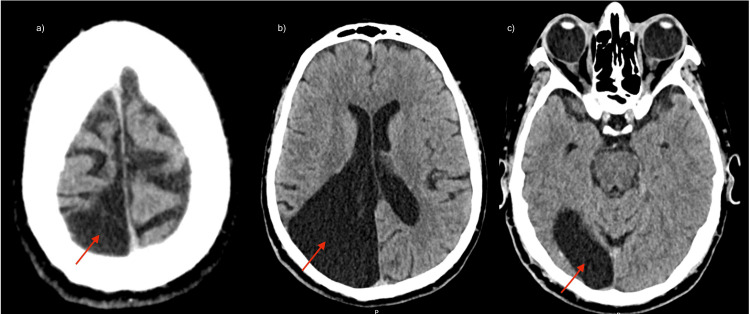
Axial brain computed tomography images showing a large right parieto-occipital arachnoid cyst. a–c: large cystic lesion (arrows) with attenuation similar to cerebrospinal fluid, showing smooth and well-defined margins, located in the right parieto-occipital region.
